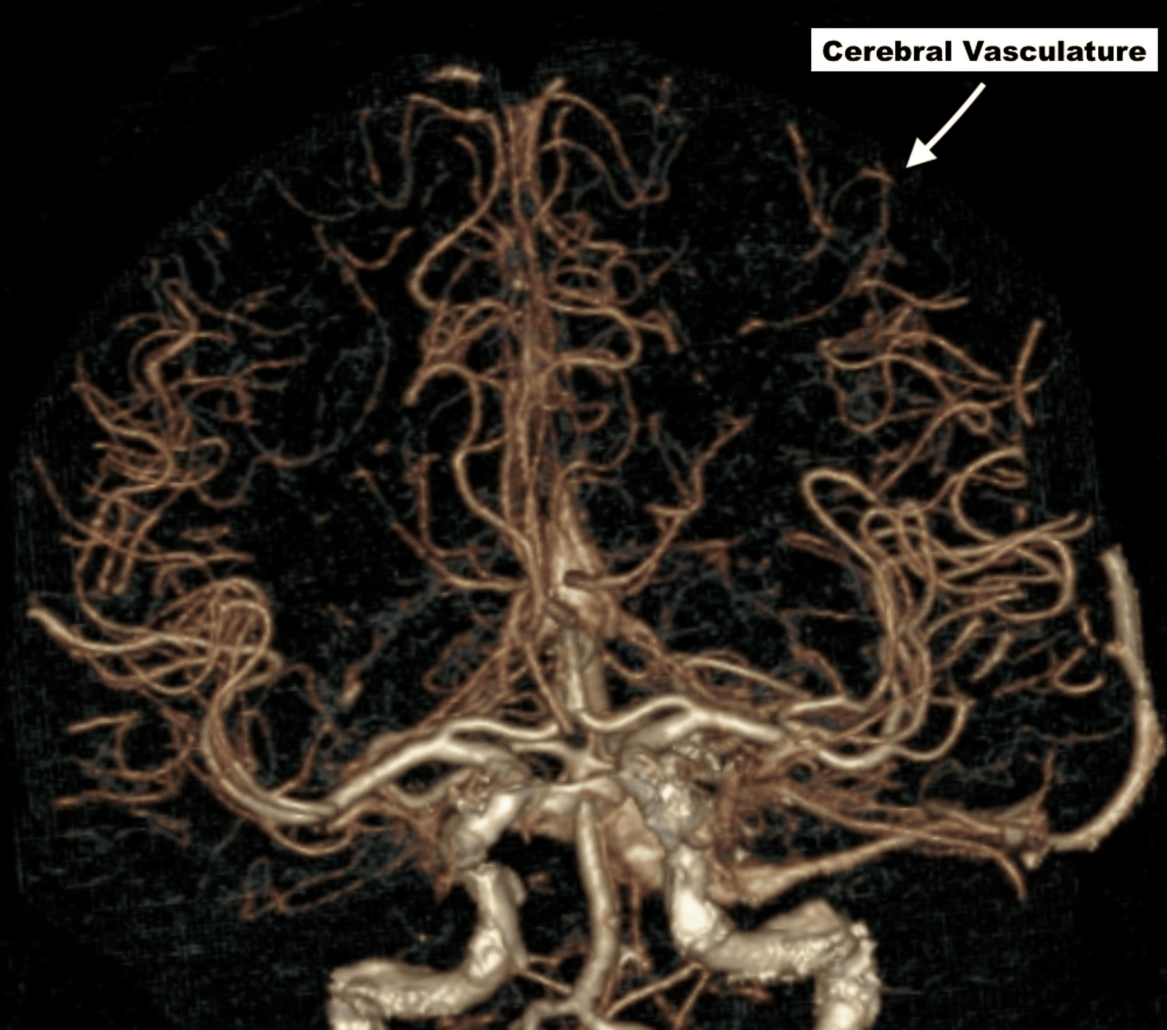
CTA brain (axial) showing absence of occlusion in the large cerebral vessels (cerebral vasculature) CTA: computed tomography angiogram
